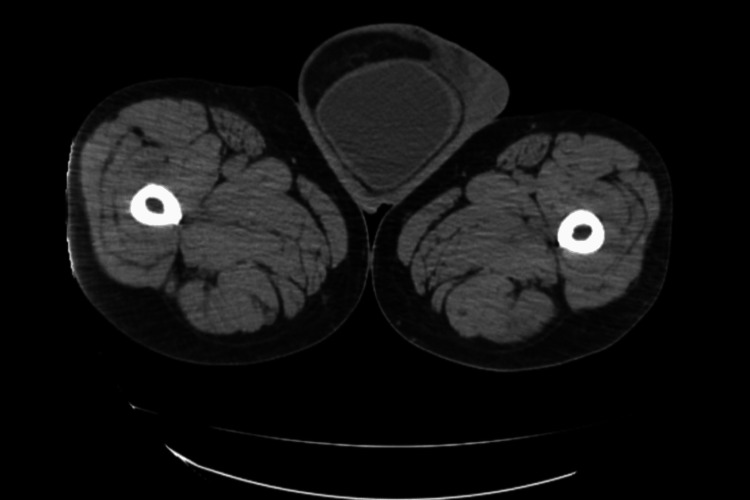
Axial view on CT demonstrating bladder in the right hemiscrotum.
